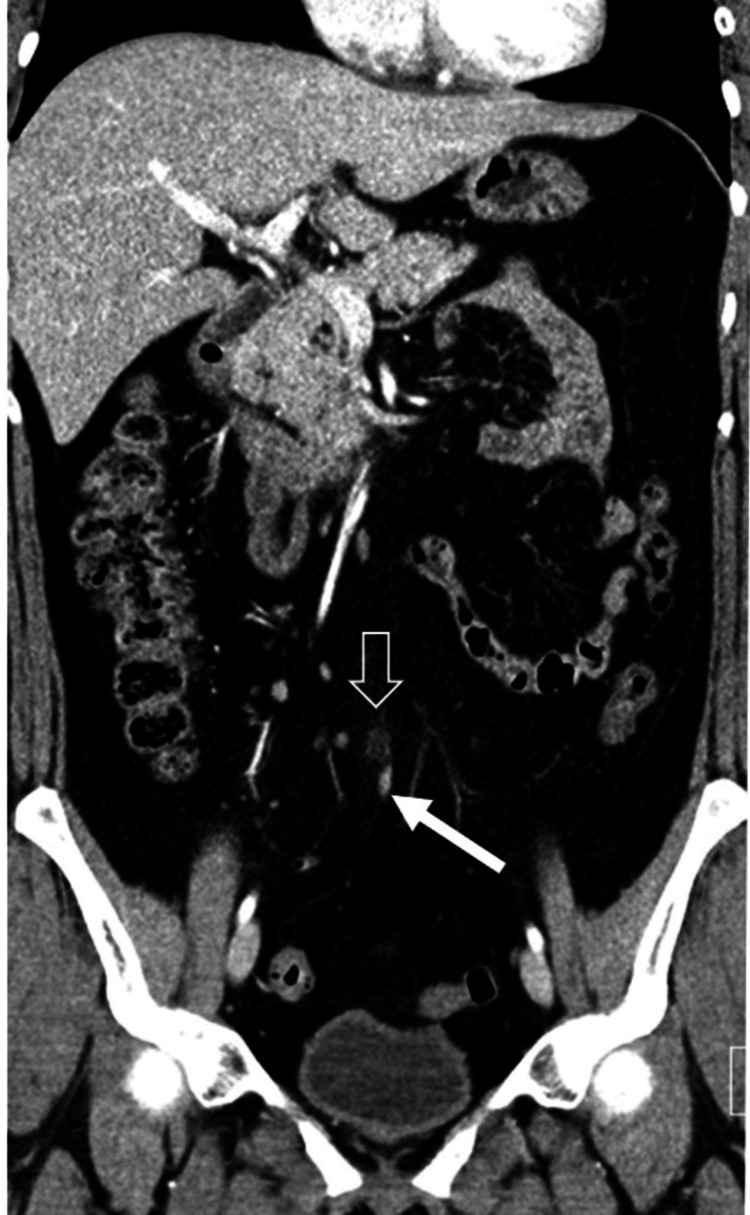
CT of the abdomen and pelvis with contrast (coronal view) showing mild focal dilation at the appendiceal tip with subtle periappendiceal fat stranding, suggesting early-stage tip appendicitis (transparent white arrow). The remainder of the appendix appears unremarkable (solid white arrow).
